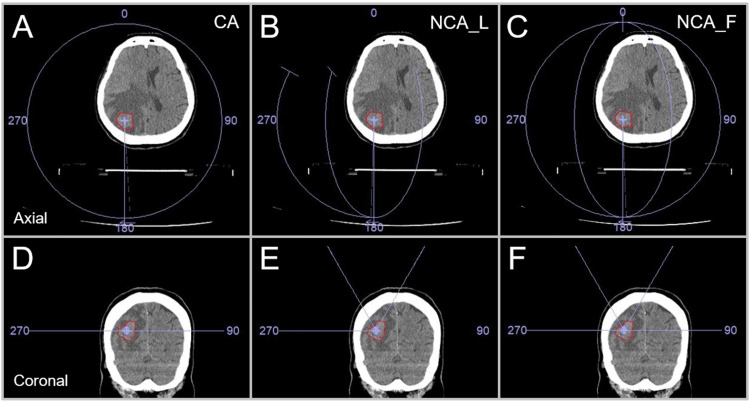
Three different arc arrangements compared. The images show head computed tomography (CT) images of a patient harboring a single brain metastasis (BM) in the right parietal lobe (A-F); the location of a gross tumor volume (GTV), the irradiation isocenter position, and three arc arrangement patterns (A, D; B, E; C, F); axial views (A-C); and coronal views (D-F). The three arc arrangements consist of two coplanar arcs (CAs) alone with to-and-fro rotations of 360º and each collimator angle of 0 and 90º (A, D); one coplanar arc (CA) and two non-coplanar arcs (NCAs) with each arc rotation of 120º to minimize the beam path lengths and the collimator angle setting of 0, 45, and 90º (B, E); and one CA with 360º rotation and the collimator angle of 0º and two NCAs with each 180º rotation and the collimator angles of 45 and 90º (C, F). The couch was rotated 60º clockwise and counterclockwise so that NCAs trisected the cephalad hemisphere (B, C, E, F). CA: coplanar arcs; NCA_L: non-coplanar arcs with limited rotations; NCA_F: non-coplanar arcs with full rotations.
